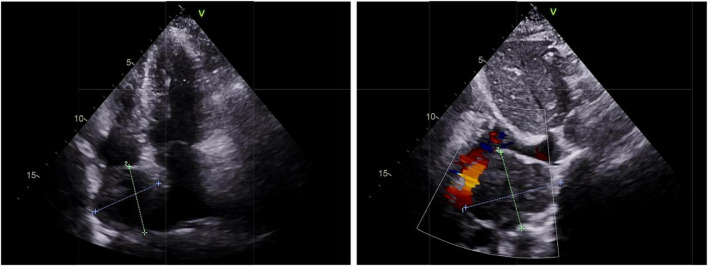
Echocardiography of cardiac paraganglioma. An enlarged left atrium and a hypoechoic mass of about 4.6 cm × 4.6 cm at the top of the right atrium near the superior vena cava was found with clear borders and uniform internal echogenicity, as well as the compression of the right atrium and the superior vena cava.
